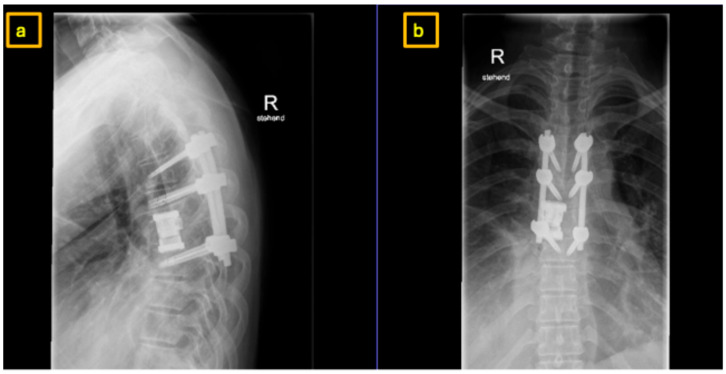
Postoperative X-Ray at 3-month follow-up demonstrating hemi-vertebral body replacement at T7 right and posterior spinal fusion from T5 to T8: (a) sagittal view, (b) coronal view.
